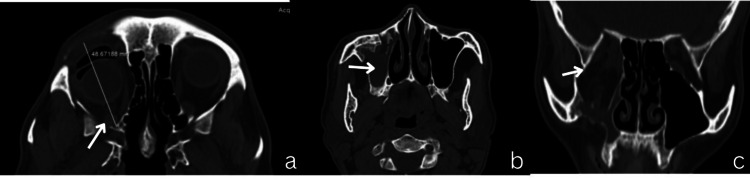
Preoperative computed tomography pictures of the patient. Axial and coronal sections of the CT scan showing enophthalmos, fracture of the infraorbital rim, and retraction of maxillary sinus walls
